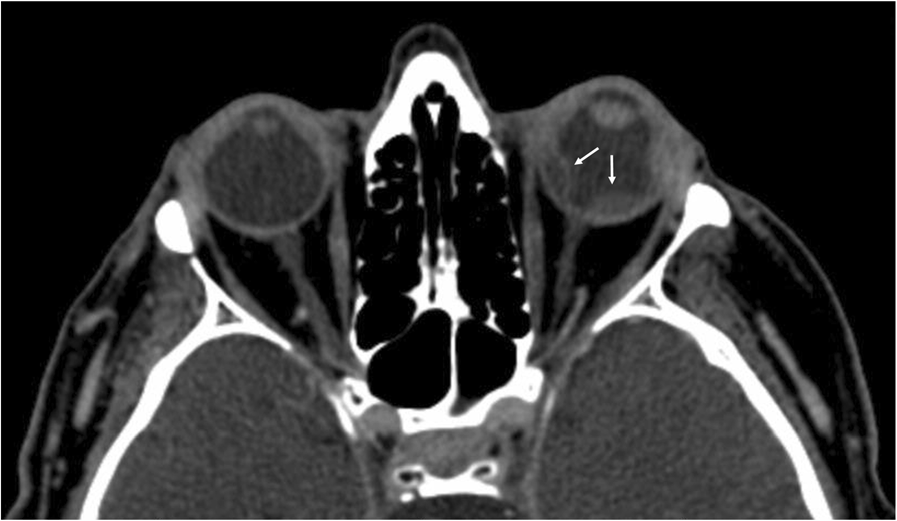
Computed tomography of the orbits. Bilateral anterior scleritis, and retinal detachment in the left eye (arrows)
